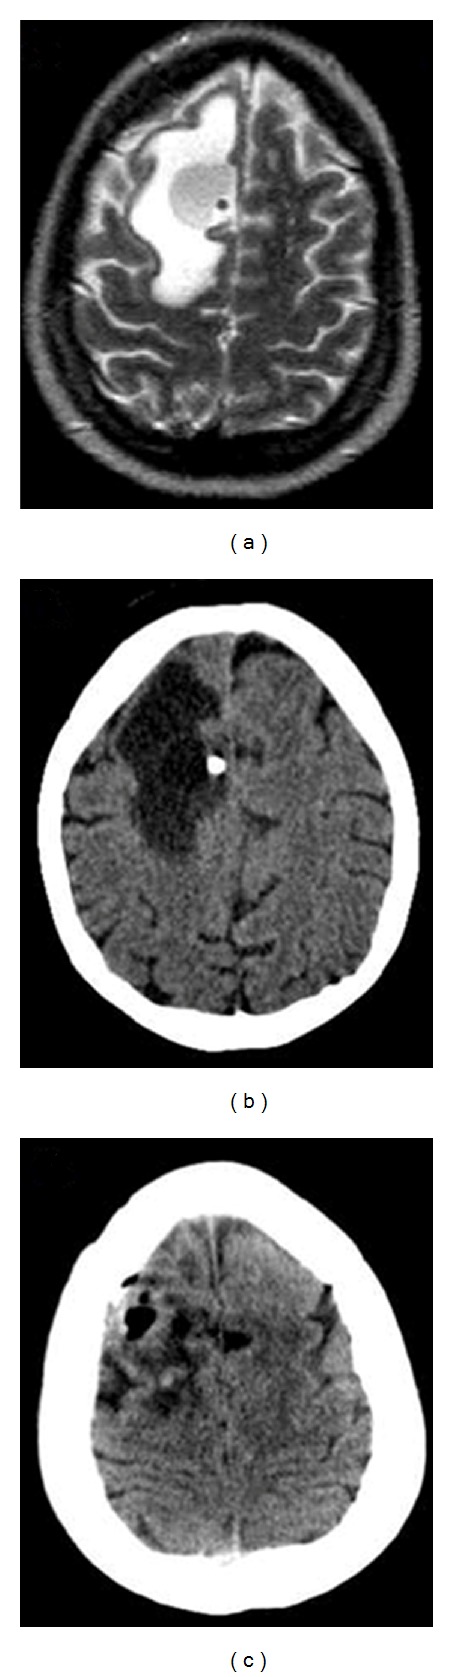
Patient B's imaging: (a) T2 MRI of the brain on admission. (b) CT of the brain on admission. (c) CT on postoperative day two, which shows resolution of the cyst and the white matter hypodensity.
